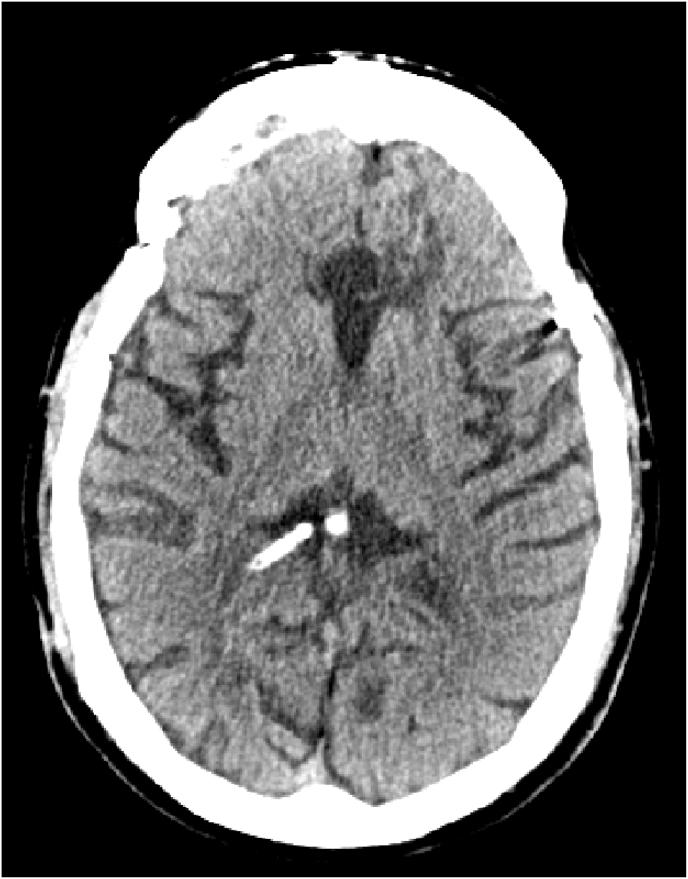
Control CT scan 1 month.
